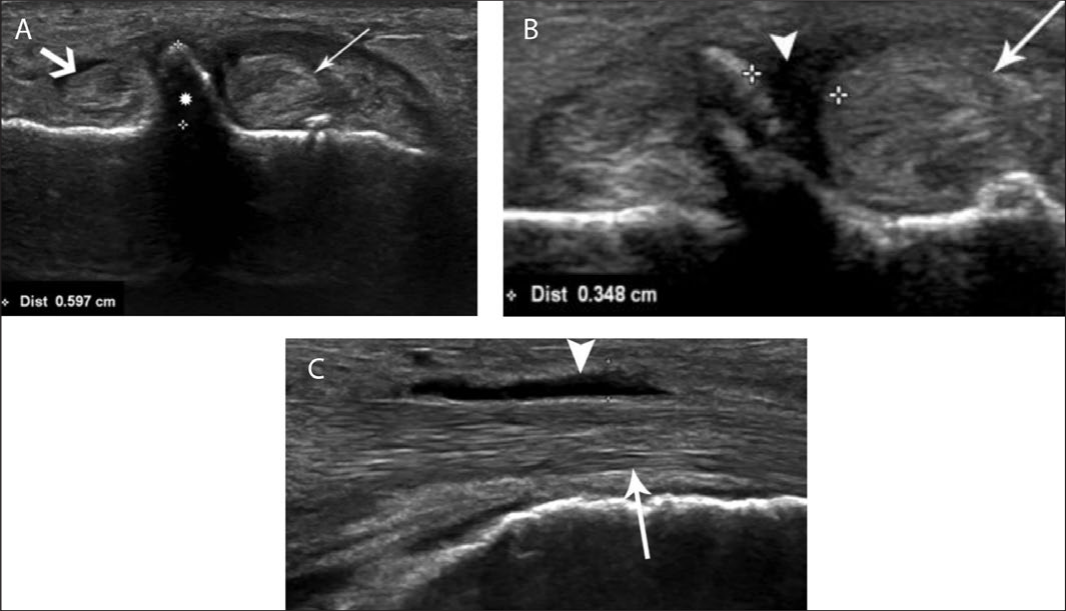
Hypertrophic TP and grade 1 tenosynovitis of the peroneus longus in a 67-year-old male. Oblique coronal (a and b) and longitudinal (c) US. Oblique coronal US (A) Reveals a hypertrophic TP (white asterisks) with a measured width of 6 mm located between the peroneus longus (thin white arrow) and peroneus brevis (thick white arrow). Note also surrounding effusion in the tendon sheath of the PL (white arrowhead) (B). On longitudinal ultrasound imaging (C) Thickening of the peroneus longus (thin white arrow) is seen with surrounding fluid (white arrowhead).
